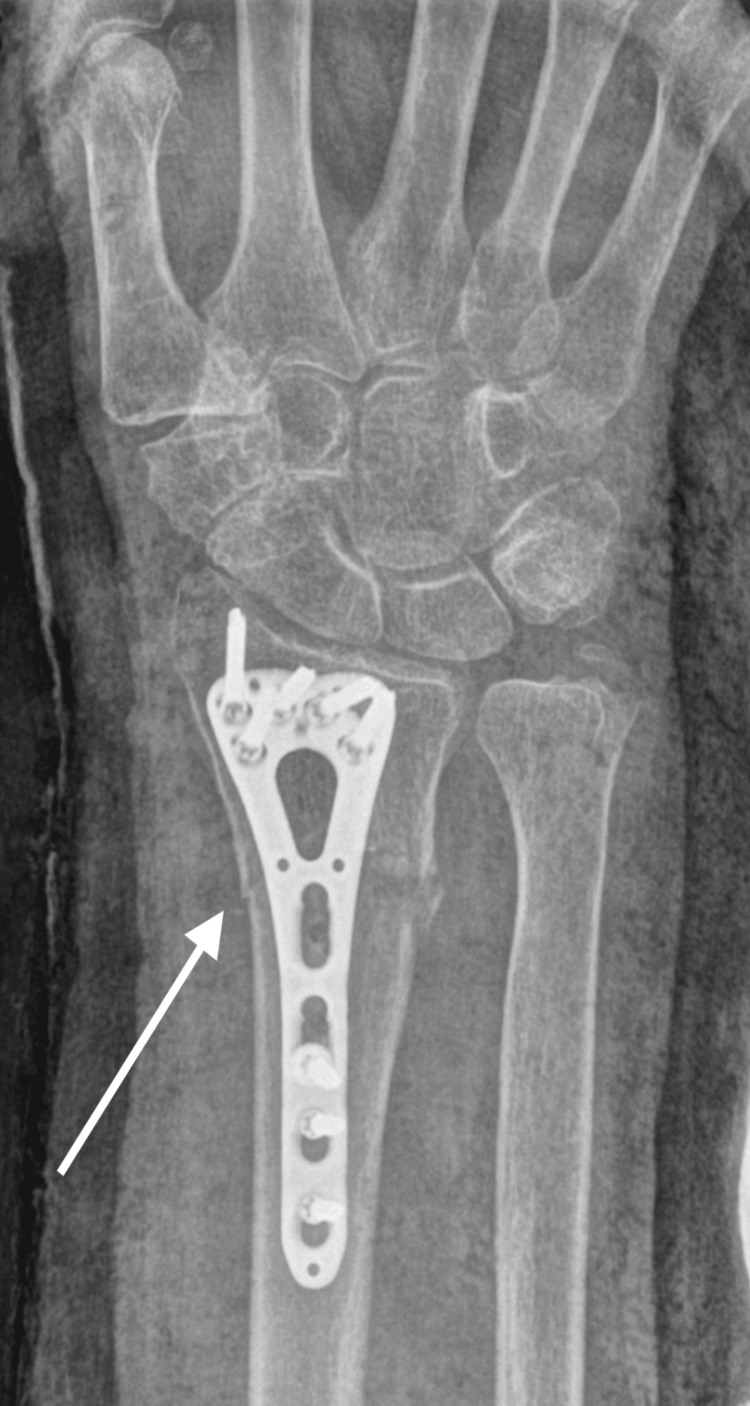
X-ray after bended plate removal and new plate insertion, anterior posterior view
